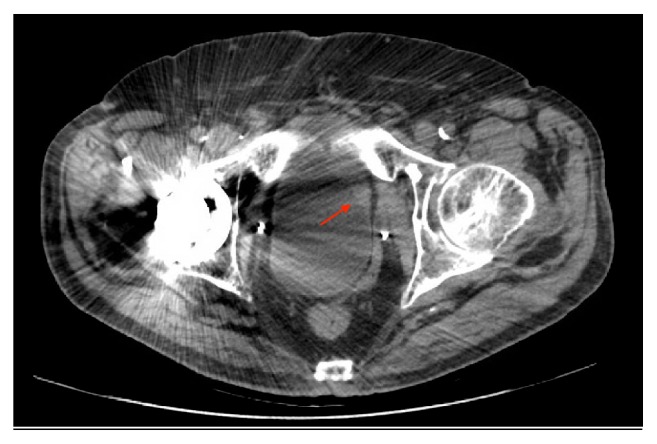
CT imaging with focal mural thickening involving the left anteroinferior neobladder.
